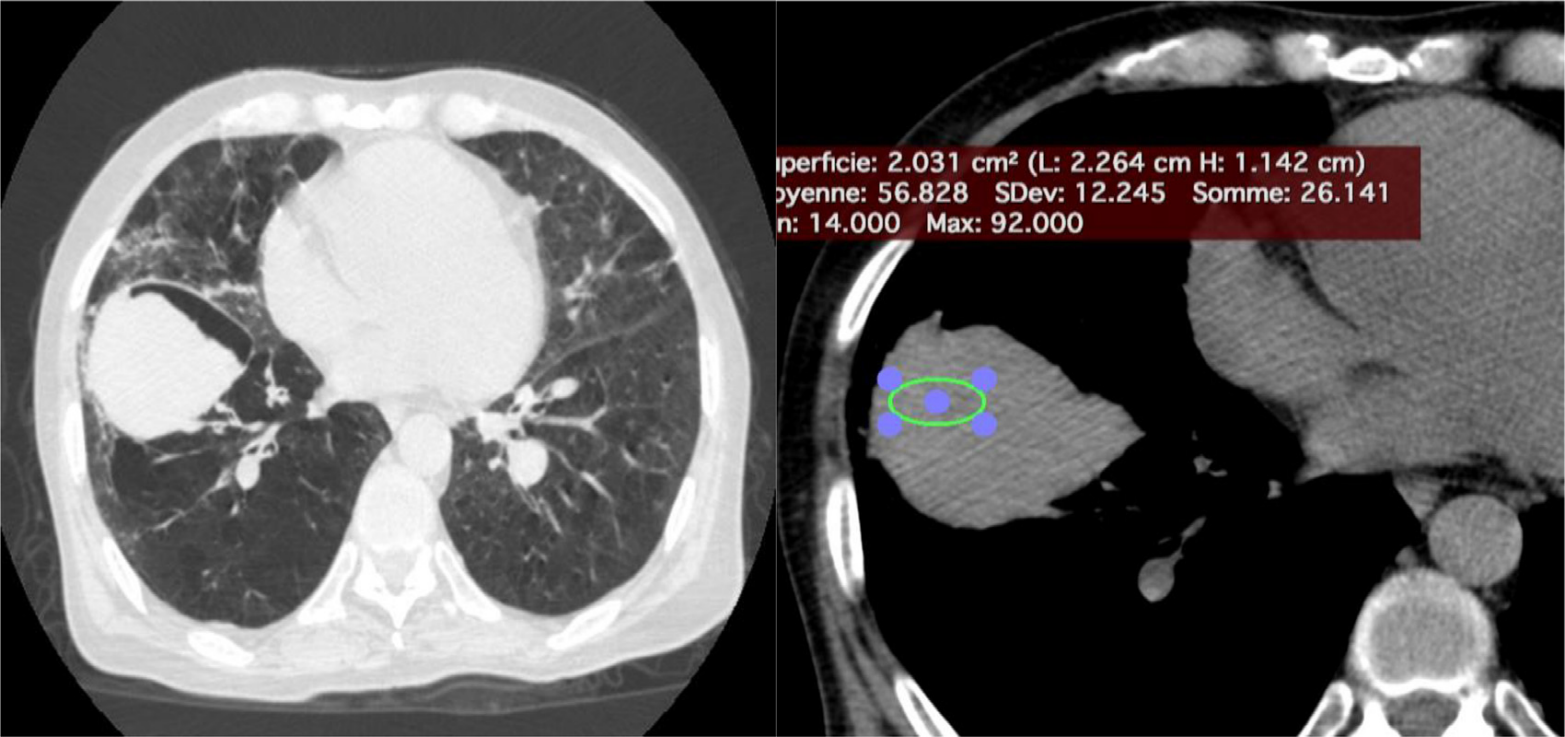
thoracic CT scan without and with contrast in mediastinal and parenchymal windows: emphysematous bulla in the middle lobe with hyperdense hemorrhagic content, noting the presence of surrounding ground-glass opacities corresponding to alveolar hemorrhage.
